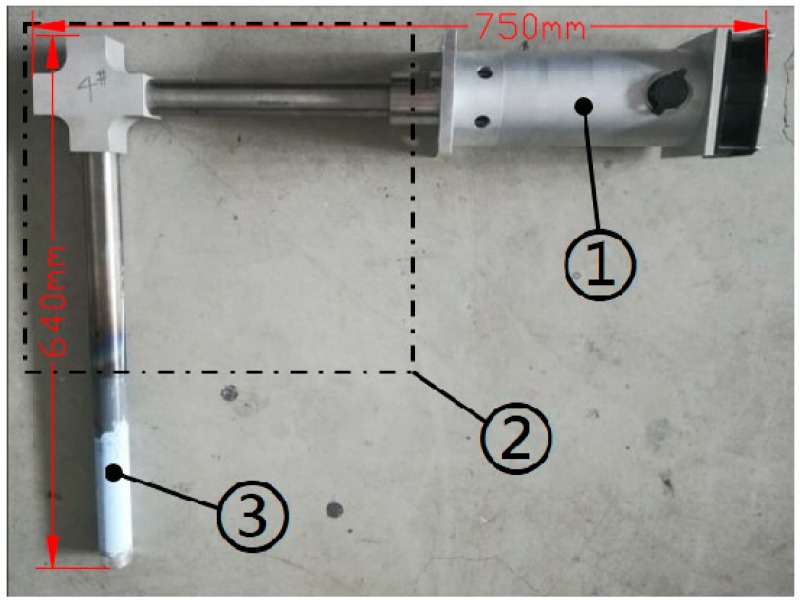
Structure of ultrasonic guided wave device.
